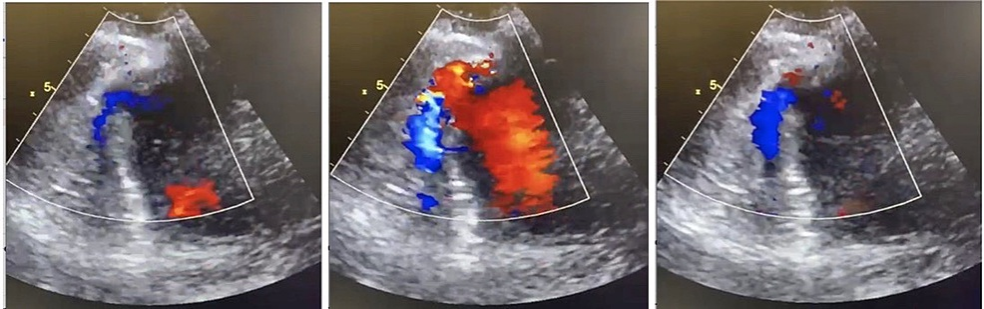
Transthoracic echocardiogram (ECHO) with Doppler colour flow demonstrating ventricular septal defect (VSD)
